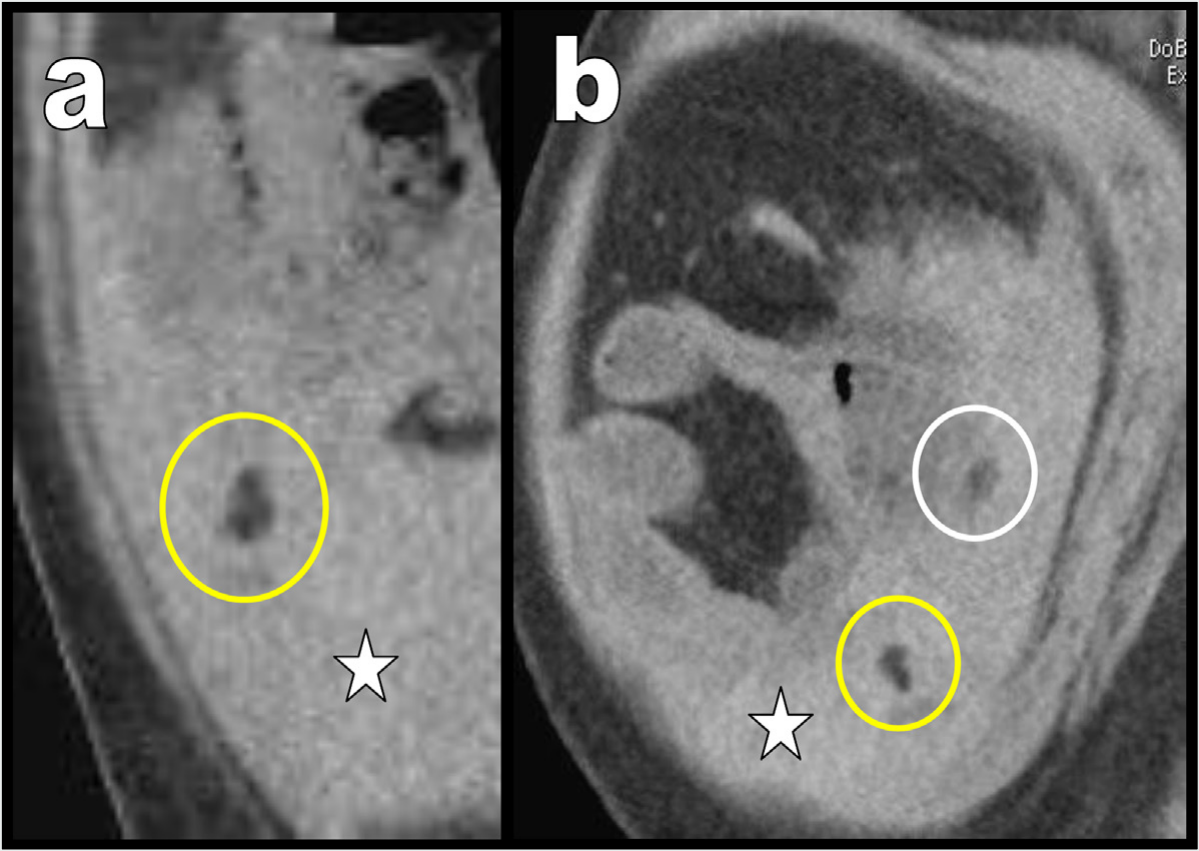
Two coronal CT oblique views of the right iliac fossa (a & b) showing small lipomatous masses (yellow and white circles) trapped in fresh blood (white star).
